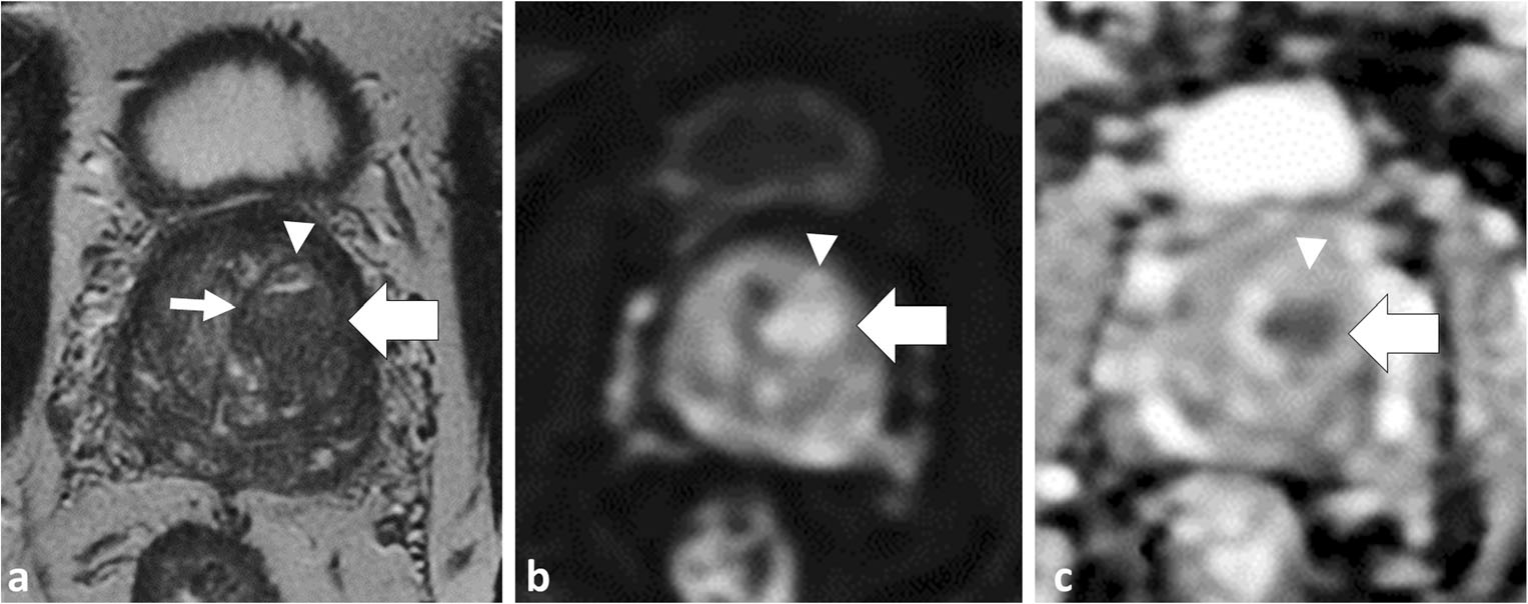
A 58-year-old patient with low-volume, low Gleason score 3 + 3 = 6 tumour in the right mid-peripheral zone undergoing MRI prior to repeat biopsy because of rising PSA. Axial T2W TSE (a) image demonstrates a low T2 SI nodule in the left TZ (*thick white arrow*). The nodule is predominantly homogeneously of low T2W SI but demonstrates a small focus of cystic change anteriorly (*arrowhead*). Note that the nodule is round and demonstrates a continuous low T2W SI rim (*thin white arrow*). The nodule demonstrates restricted diffusion, increased SI on trace b1000 mm²/s EPI (b) and low SI on the ADC map (c) (*thick white arrows*). Note that cystic change demonstrates T2 shine-through (*arrowhead*). Imaging findings are characteristic of a mixed but predominantly stromal BPH nodule
